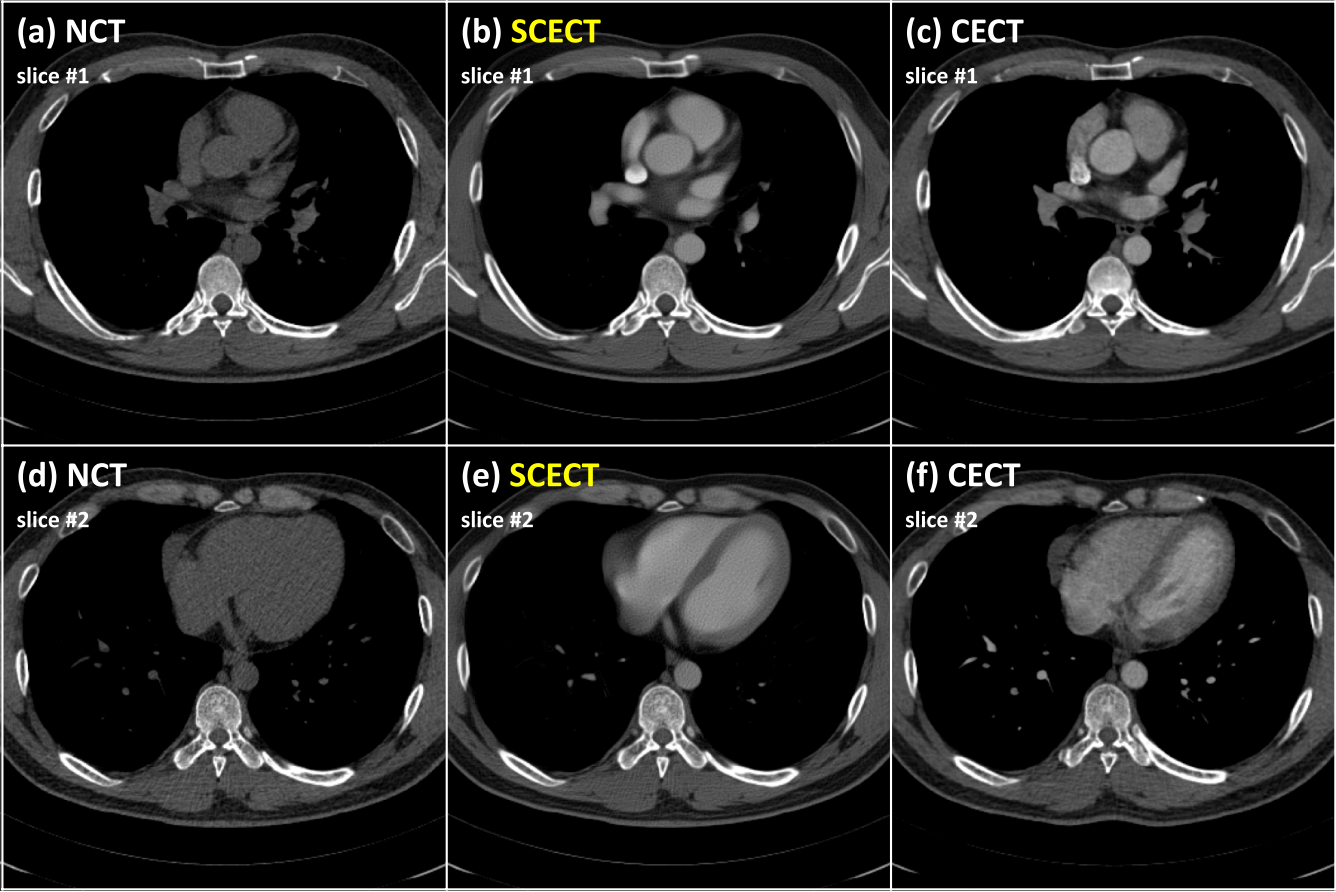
Representative images of non-contrast computed tomography (NCT) (a, d), synthetic contrast-enhanced CT (SCECT) (b, e), and contrast-enhanced CT (CECT) (c, f) of testing datasets. The upper and lower rows indicate different slices of the test datasets
